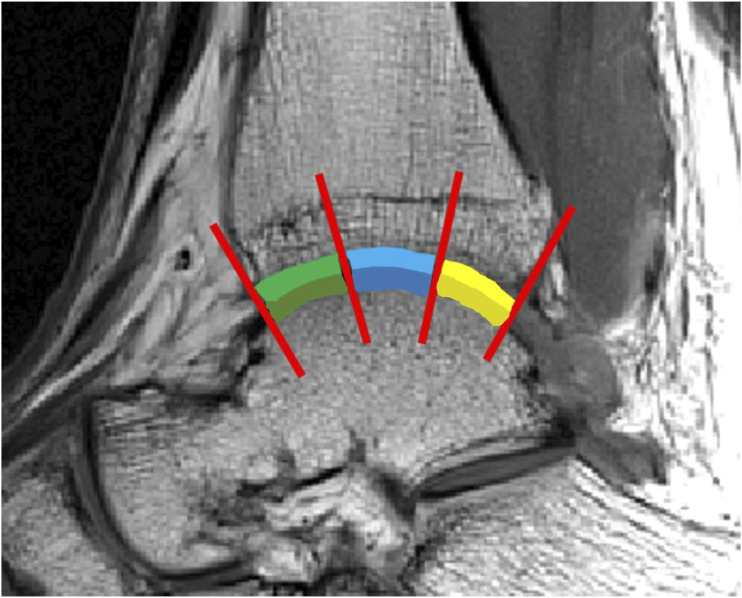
Sagittal T2-weighted image of ankle joint from a 20-year-old male (stable fracture). Each of the studied three regions is indicated by their respective color: green = anterior region, blue = middle region, yellow = posterior region. Lighter and darker color indicate tibial and talar cartilage, respectively.
